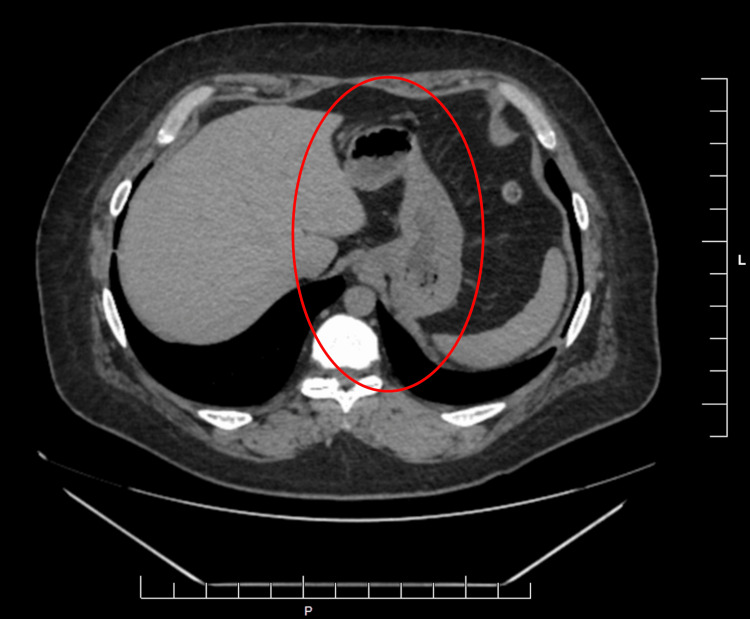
Axial contrast-enhanced computed tomography (CT) of the abdomen and pelvis. The axial image at the level of the gastric body demonstrates mild gastric distension with a visible air-fluid level (as depicted by the red circle). There is no evidence of focal mural thickening, suspicious masses, or mechanical gastric outlet obstruction. Surrounding inflammatory changes, such as fat stranding or free fluid, are notably absent.
